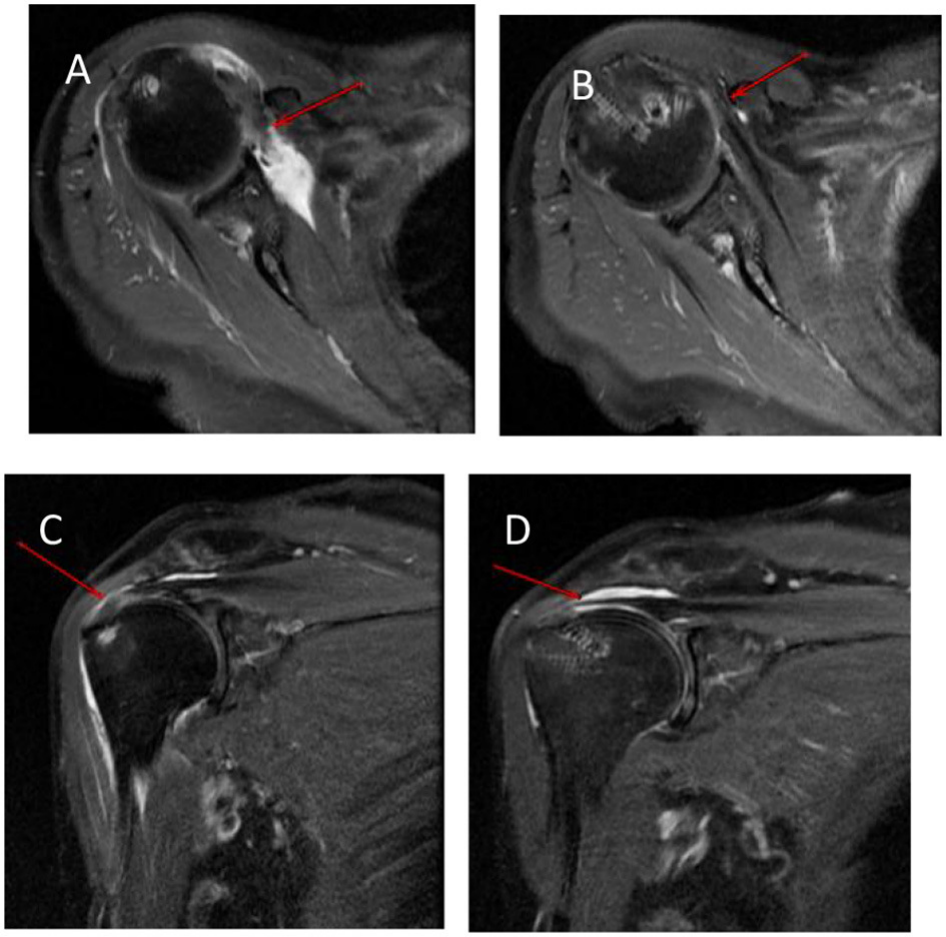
Preoperative and postoperative MRI scans of the right shoulder in a 71-year-old female patient. (A) An axial T2-weighted MRI scan shows a chronic subscapularis tear (arrows) preoperatively. (B) Complete structural integrity of the repaired subscapularis tendon (arrows) is shown on axial T2-weighted MRI at 12 months postoperatively. (C) A coronal T2-weighted MRI scan shows a combined supraspinatus tear. (D) Complete structural integrity of the repaired supraspinatus tendon is shown on coronal T2-weighted MRI at 12 months postoperatively.
